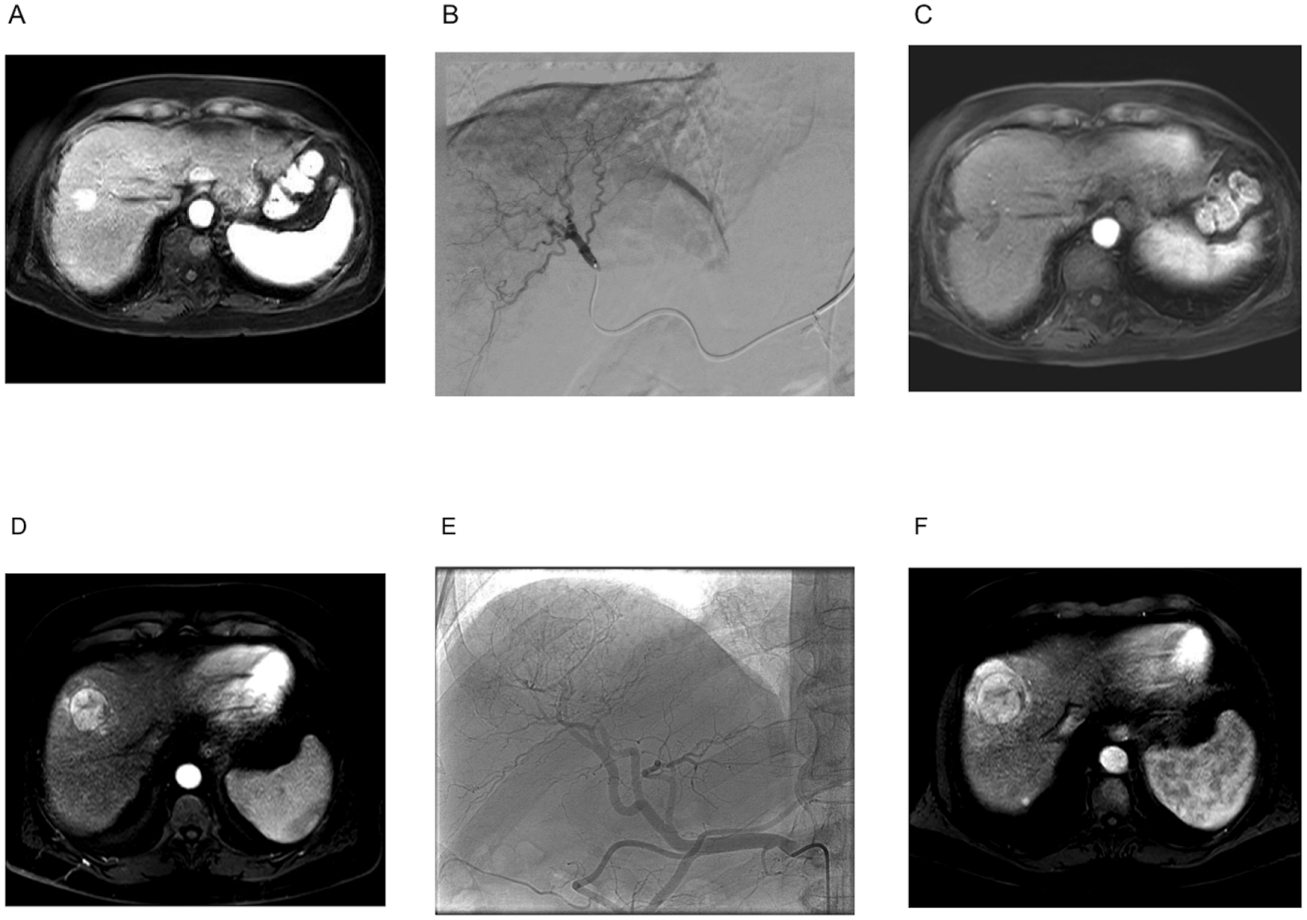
Example magnetic resonance imaging before and after local ablative therapy. A) MRI of an HCC before treatment of a responding patient. HCC shows contrast enhancement in T1 sequence (arrow). B) Angiography image taken during TACE. HCC lesion is pooling arterial flow (arrow). C) Follow-up MRI of the responder patient. The lesion only shows ablation lesion after RFA/TACE without contrast enhancement. D) MRI of an HCC before treatment of a non-responding patient. HCC shows contrast enhancement in T1 sequence (arrow). E) Angiography image taken during TACE. HCC lesion is pooling arterial flow (arrow). F) Follow-up MRI of the responder patient. The contrast-enhancing lesion shows increase in size (arrow).
